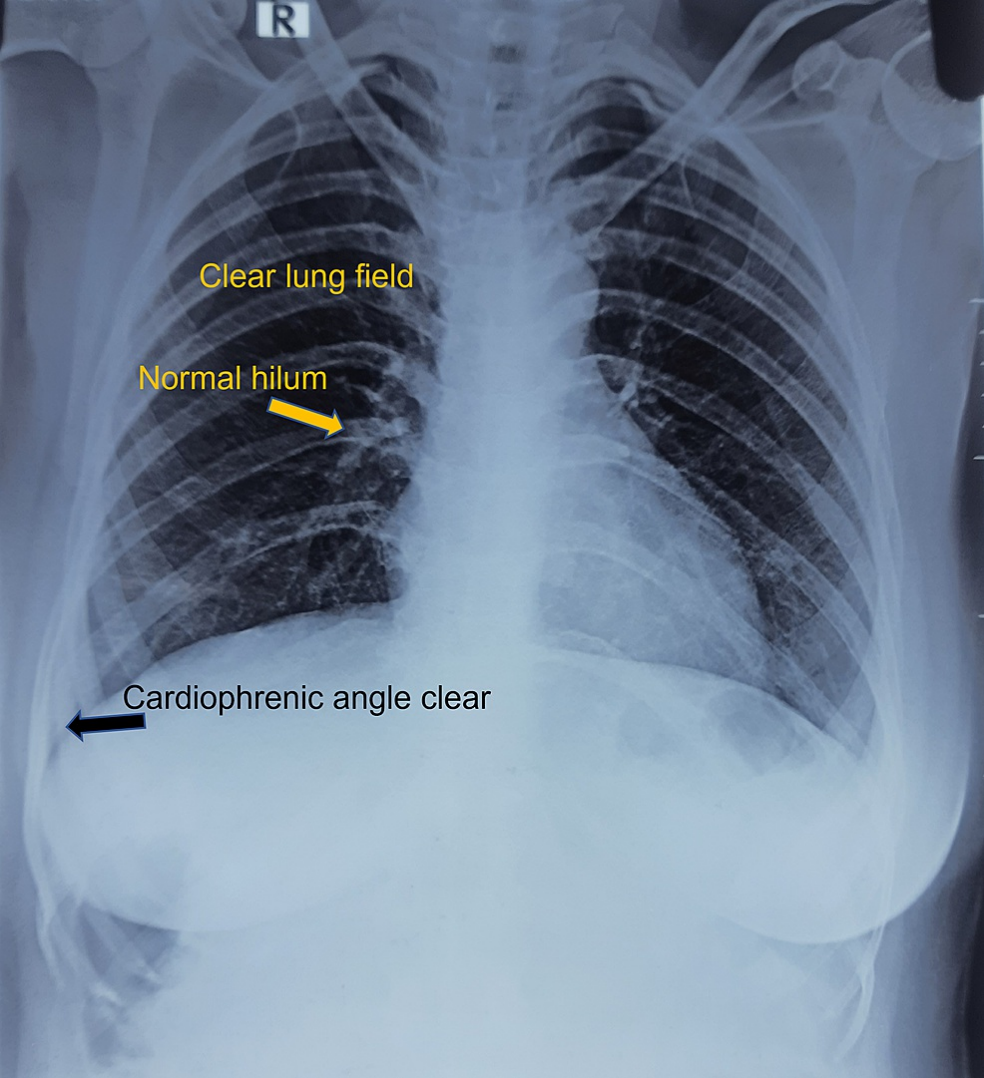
Chest X-ray (PA view) showing clear lung fields PA: posteroanterior.
